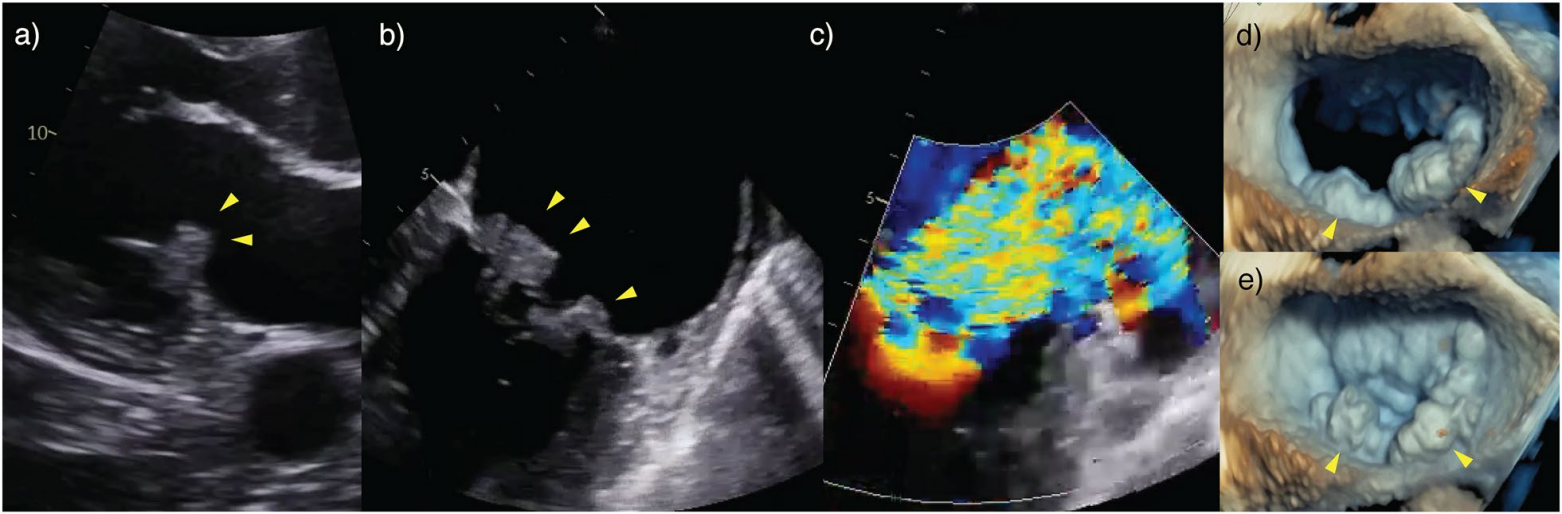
Case 1—Infective endocarditis. Vegetations highlighted with arrows on the a) parasternal long-axis (PLAX) TTE view and (b) mid-oesophageal commissural TOE view; c colour Doppler showing severe MR; d (diastole) and e (systole) demonstrates a 3D TOE image of the mitral valve vegetations (arrows)
